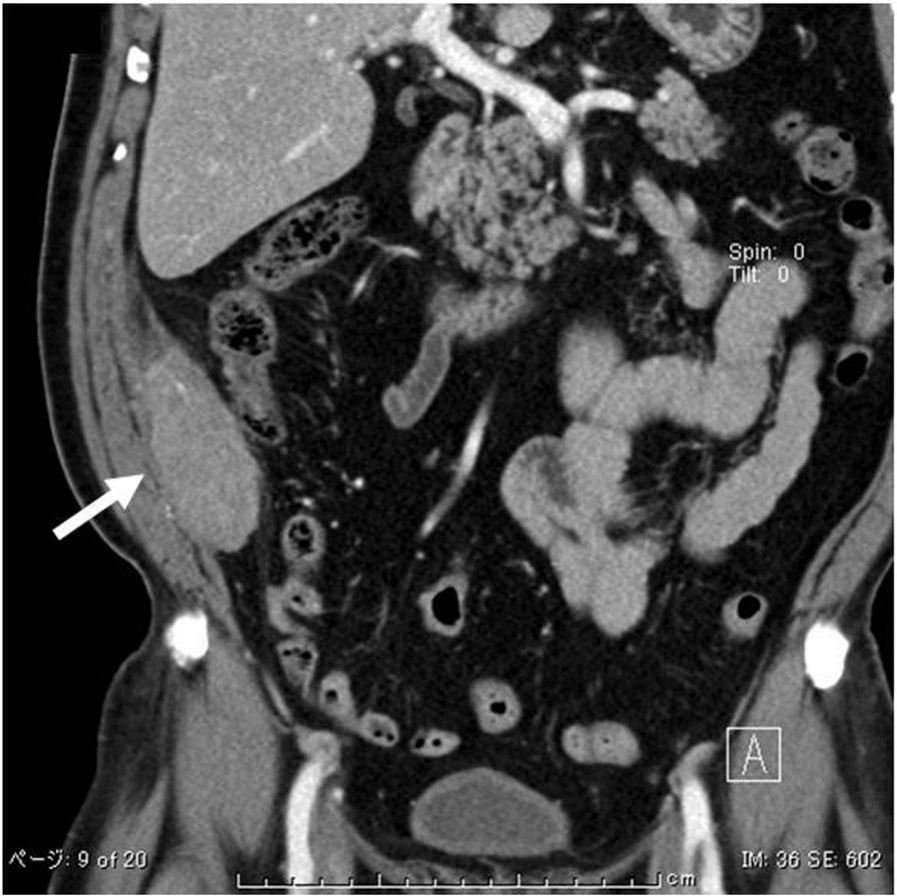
Helical computed tomography (CT) indicating a solid mass in the abdominal wall (arrow)
